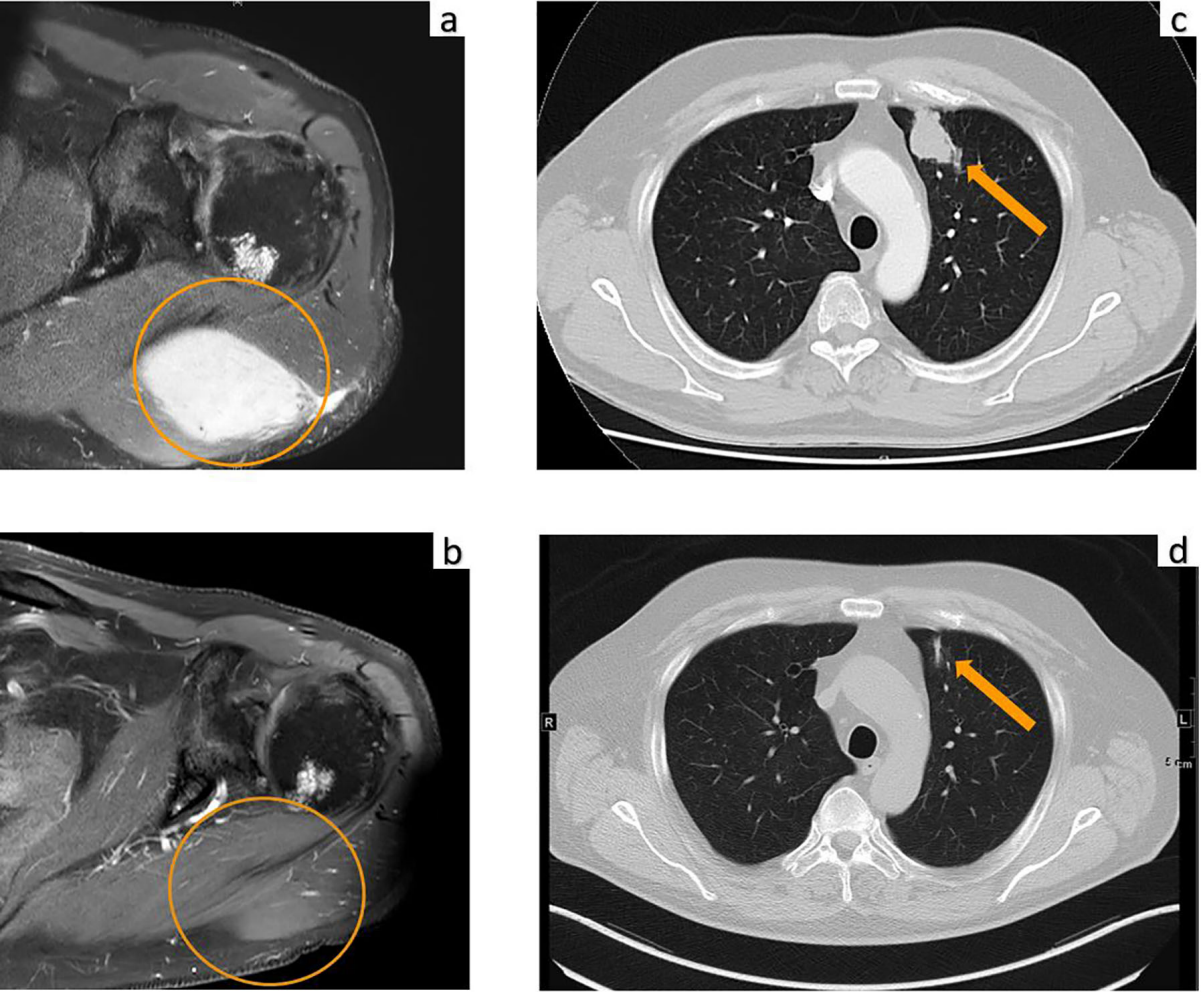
MRI of the upper left extremity: (A) Strong contrast enhancement of a 4.0 × 2.6 cm metastasis of the left deltoid muscle before initiation of immunotherapy. (B) Complete resolution of the tumor mass at three months after the first and only dose of ipilimumab plus nivolumab. Computed tomography of the chest: (C) Metastasis of the left upper lobe (arrow) before initiation of immunotherapy. (D) Complete resolution of the lung metastasis with residual dystelectasis (arrow) at three months after the first and only dose of ipilimumab plus nivolumab.
